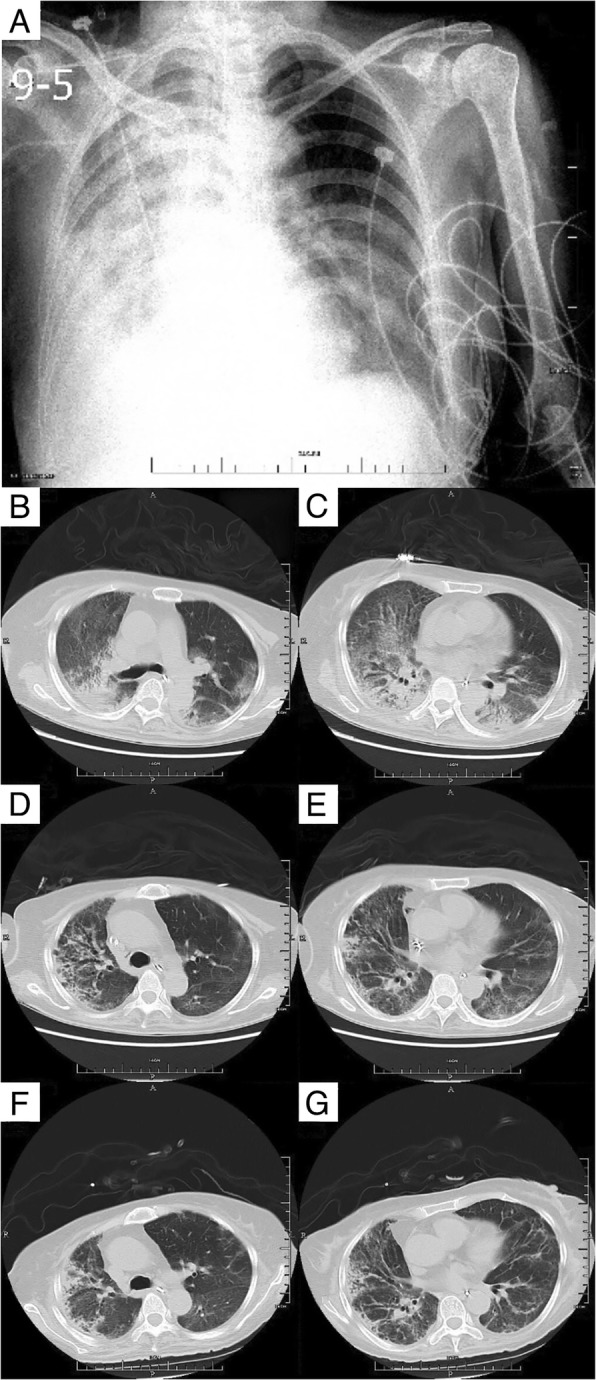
Chest X-ray and chest computed tomography findings in Case 2*,* which were examined at the time of therapeutic initiation (a), 5 days after tests for H7N9 virus turned negative, on the day of mechanical ventilation withdrawal (b and c), in the clinical course of day 31 (d and e) and on the day of being discharged (f and g)
